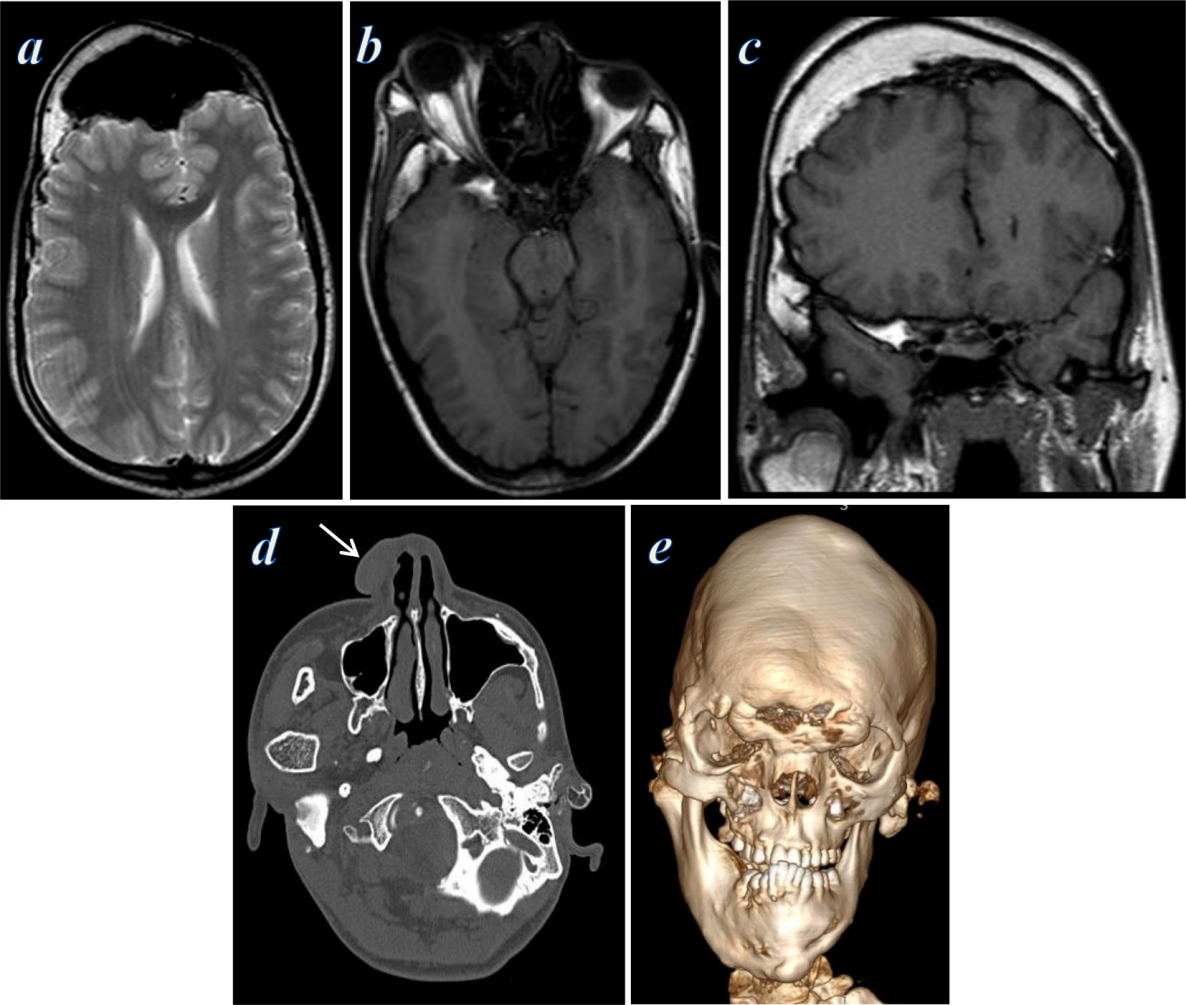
MRI and CT examination of the brain and face. (a) Axial *T*
